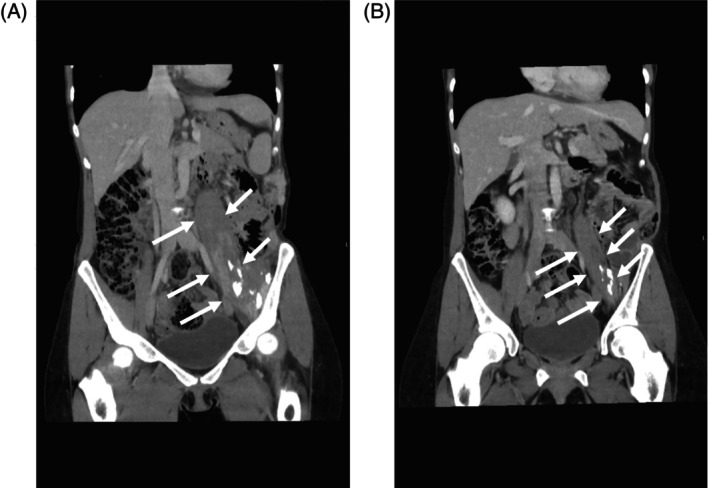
Computed tomography imaging showed inhomogeneous soft tissue formations in the psoas muscle, the iliopsoas and in the lateral paravertebral musculature (indicated by arrows) before initiation of crizotinib (A) and 5 months into therapy (B). The abdominal wall metastasis on the left paraumbilical side regressed considerably regarding soft tissue involvement. Grossly flaked calcifications and a decrease in volume, but no pathologic uptake of contrast remained in the affected muscular structures.
